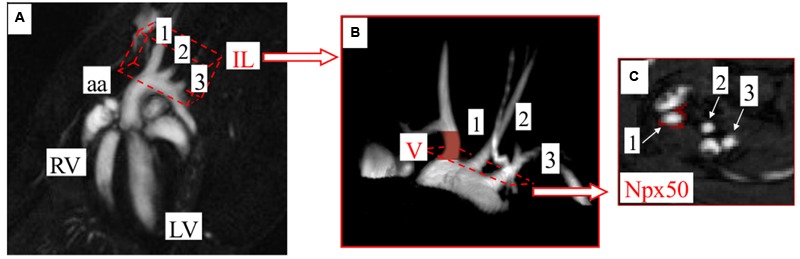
Methodology to assess endothelium-dependent response and changes in endothelial permeability in brachiocephalic artery (BCA) *in vivo* by MRI. (A) Coronal view of the heart. Image showing position of the imaging layer (IL) used for 3D imaging of the aortic arch. (B) 3D image of aortic arch acquired with the cine IntraGate^®^ FLASH 3D sequence. Endothelial function assessment, expressed as changes in the vessels volume (V) can be performed in vessels arising from aortic arch. The end-diastolic volumes of BCA were assessed prior to and 25 min after intraperitoneal Ach administration. (C) Representative images of vessels cross-sections, in which a number of pixels, for which T_1_ had changed more than 50% after CA administration (Npx50), is marked in red. BCA is indicated as 1, left carotid artery (LCA) as 2, left subclavian artery (LSA) as 3. RV, right ventricle; LV, left ventricle.
